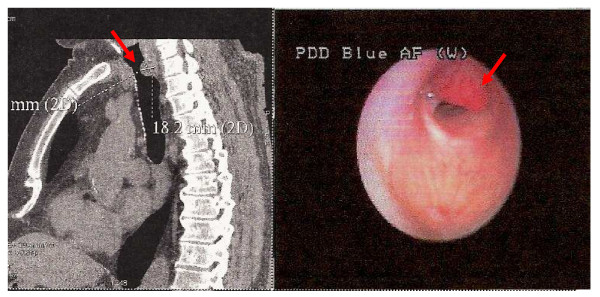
The sagittal CT-scan showed the presence of an endotracheal tumour under the glottis (left panel). Presence of a vascularised tumor of the trachea visible by bronchial endoscopy (right panel).
